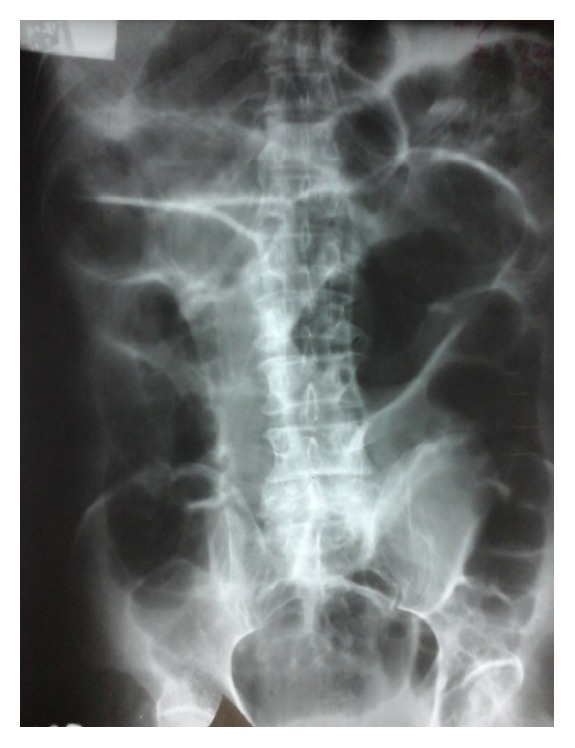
Supine X-ray abdomen showing dilated small bowel loops.
